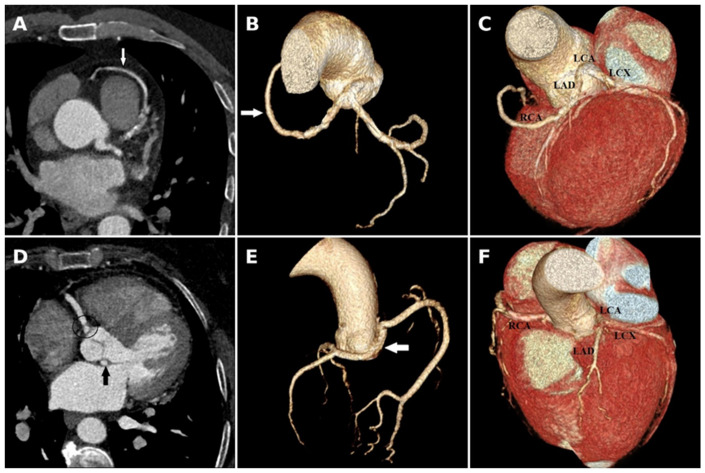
Coronary computed tomography angiography findings in two patients with single coronary artery variants. (A–C): A 67-year-old man with Lipton type L-II-A single coronary artery. (A) Axial CCTA shows the right coronary artery (RCA) originating from the mid left anterior descending artery (LAD) and coursing anterior to the pulmonary artery (arrow), consistent with a prepulmonic course. (B) Volume-rendered reconstruction demonstrating the anomalous RCA (arrow). (C) Three-dimensional overview with labeled coronary branches. (D–F): A 71-year-old woman with Lipton type R-II-P single coronary artery. (D) Axial CCTA shows the left coronary artery arising from a common trunk with the RCA (circle) and passing posterior to the aorta (arrow), consistent with a retroaortic course. (E) Volume-rendered image highlighting the anomalous left coronary origin and course (arrow). (F) Three-dimensional reconstruction showing the complete coronary anatomy with labeled vessels.
